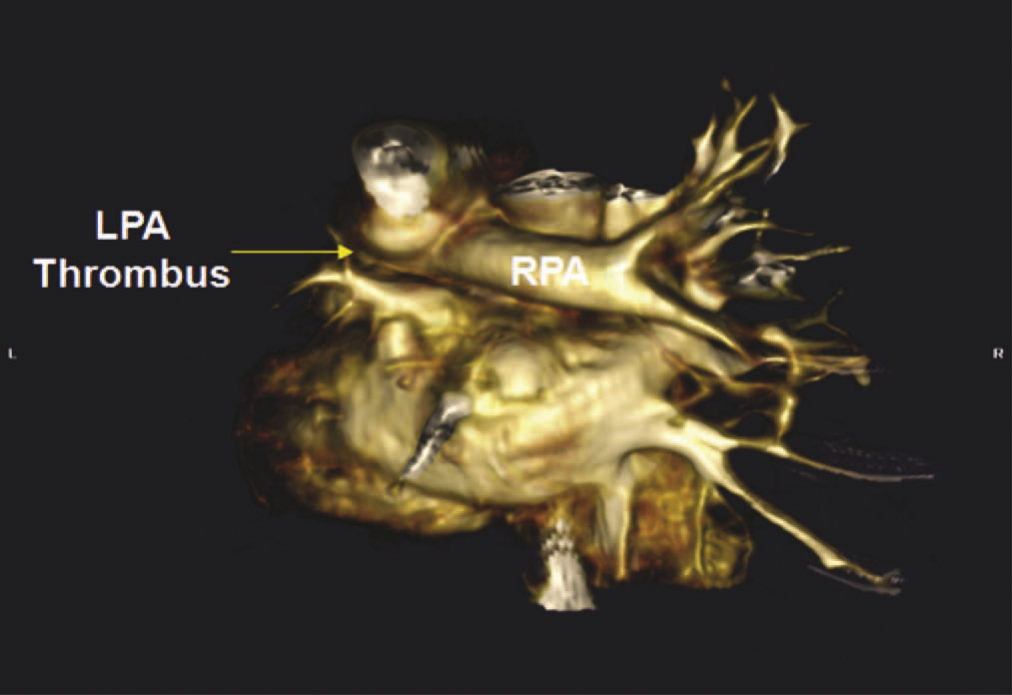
Three-dimensional view on computed tomography angiography showing a filling defect in the lumen of the left pulmonary artery (LPA) consistent with LPA thrombosis.
